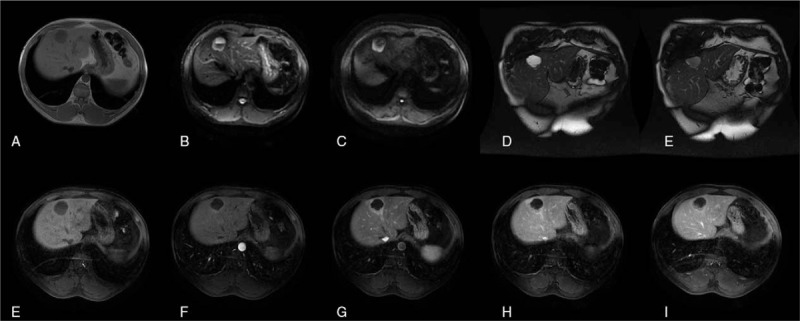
MRI results of 26 months after initial hospitalization showed that the diameter of the lesion increased from 40 mm to 60 mm. (A) Hybrid low signal on T1WI. (B) The axial T2WI lipid image showed mixed high signal. (C) DWI. (D) Coronal T2WI weighted images. (E) Coronal T2WI weighted images. (F) Enhanced anterior mask. (G) Early enhancement of arteries. (H) Late enhancement of arteries. (I) Enhanced venous phase. (J) Enhanced delayed phase.
